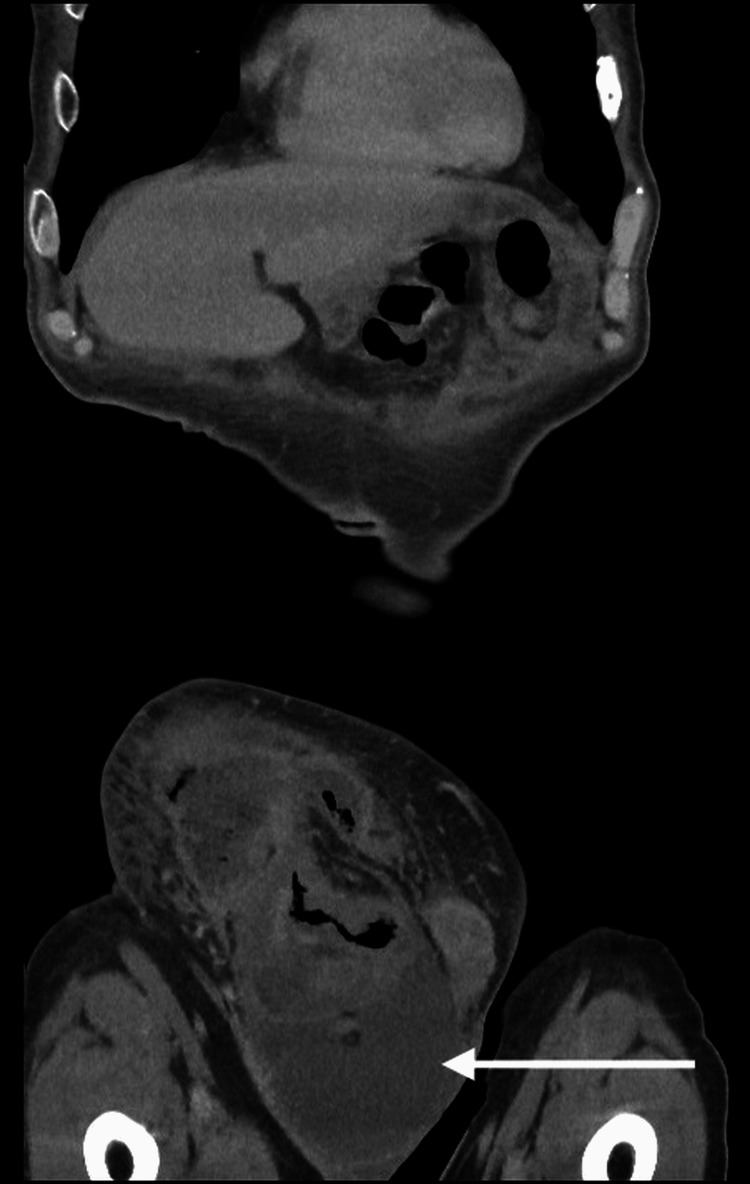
CTAP coronal plane - 2 The hydrocele CTAP: computed tomography of the abdomen and pelvis
